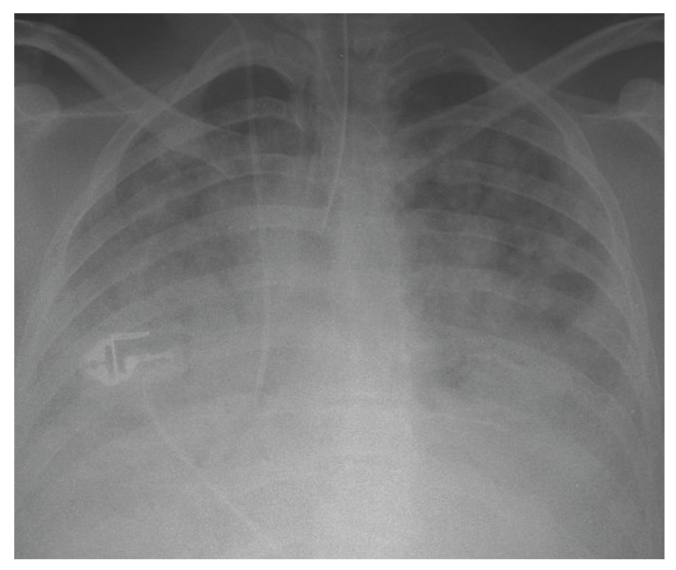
Postero anterior radiograph of the chest obtained after tracheal intubation on day 1.
